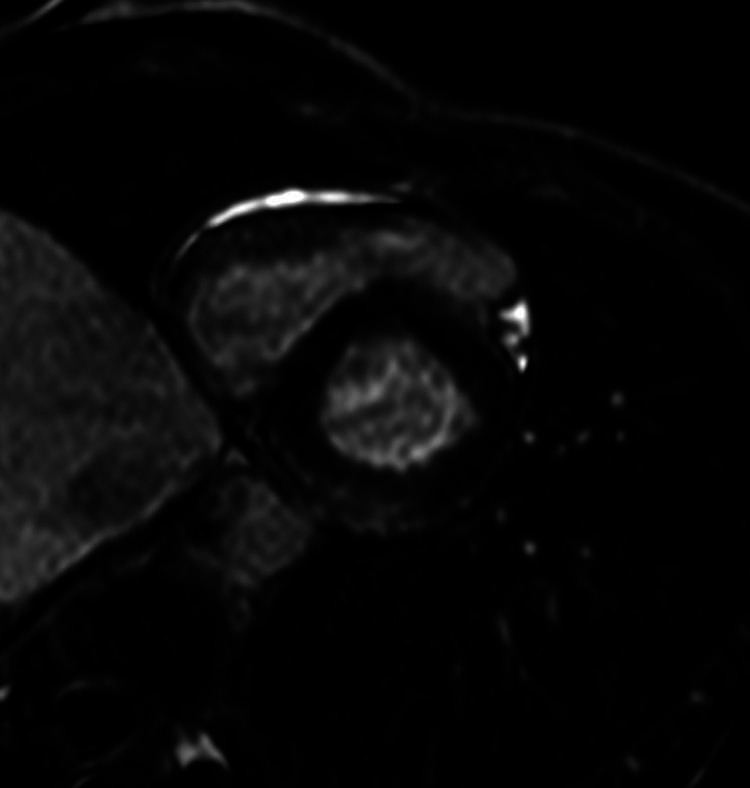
MRI image analysis. Precontrast images: on STIR T2w image areas of signal hyperintensity indicative of myocardial oedema in mid-basal region of inferior and posterior walls are observed.
